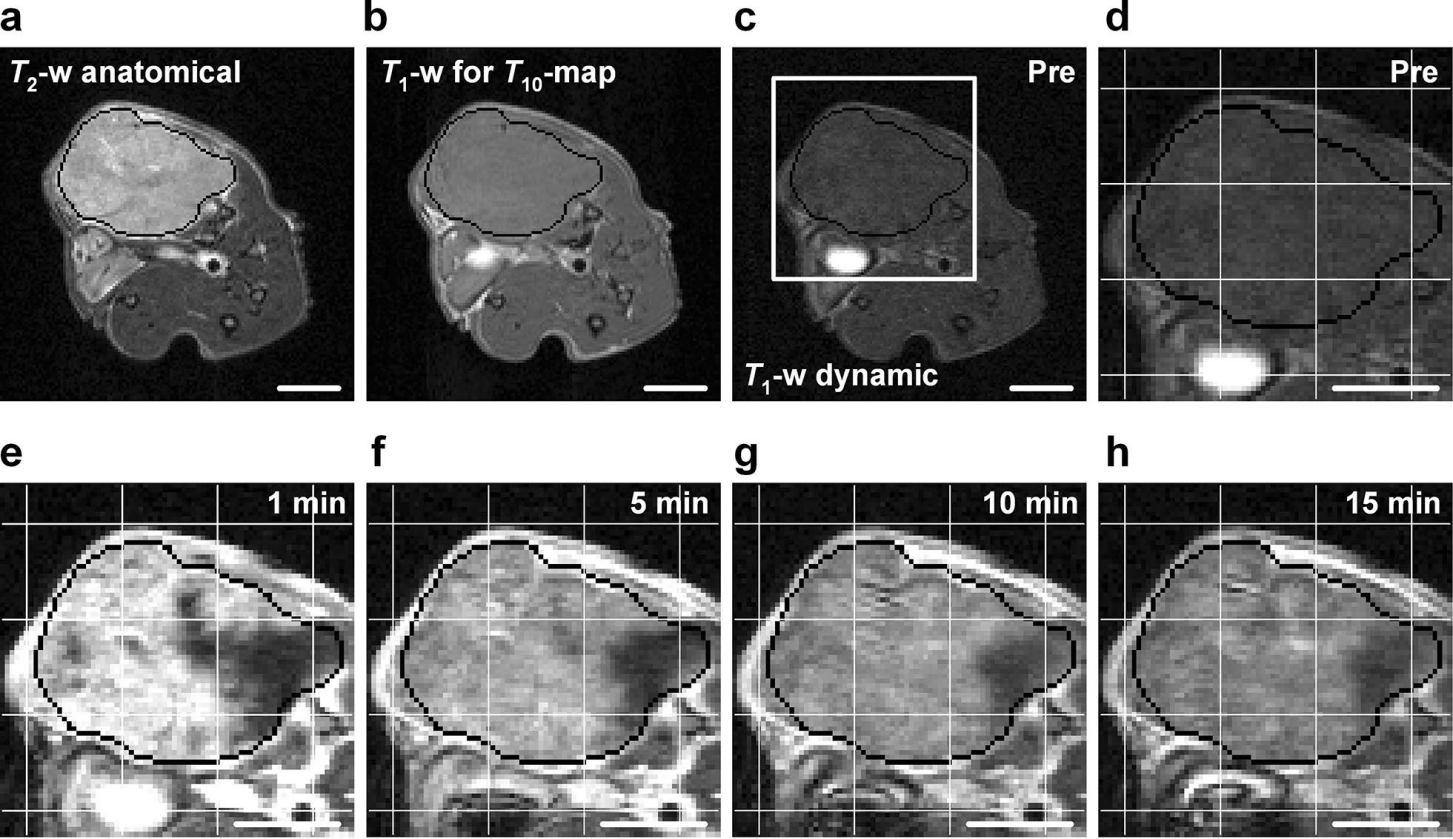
MR images of a representative tumor. *T*
